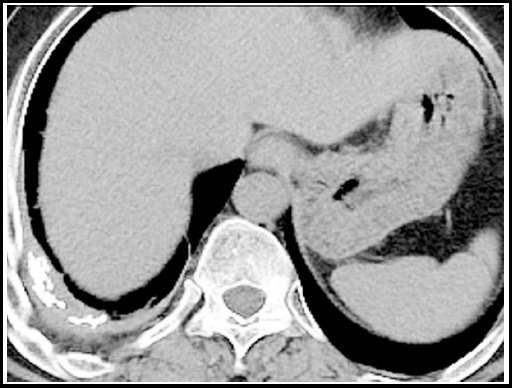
CT scan chest in mediastinal window settings shows right pleural thickenng with calcification.
